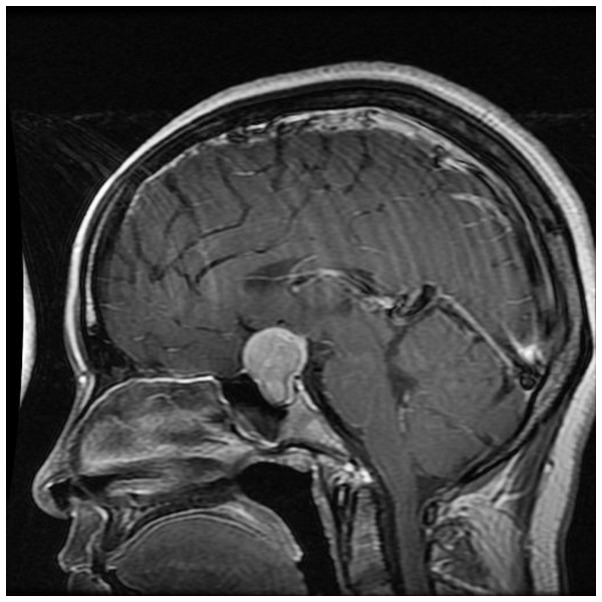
MRI showing a sellar lesion, and heterogeneous enhancement on contrast MRI. MRI, magnetic resonance imaging.
